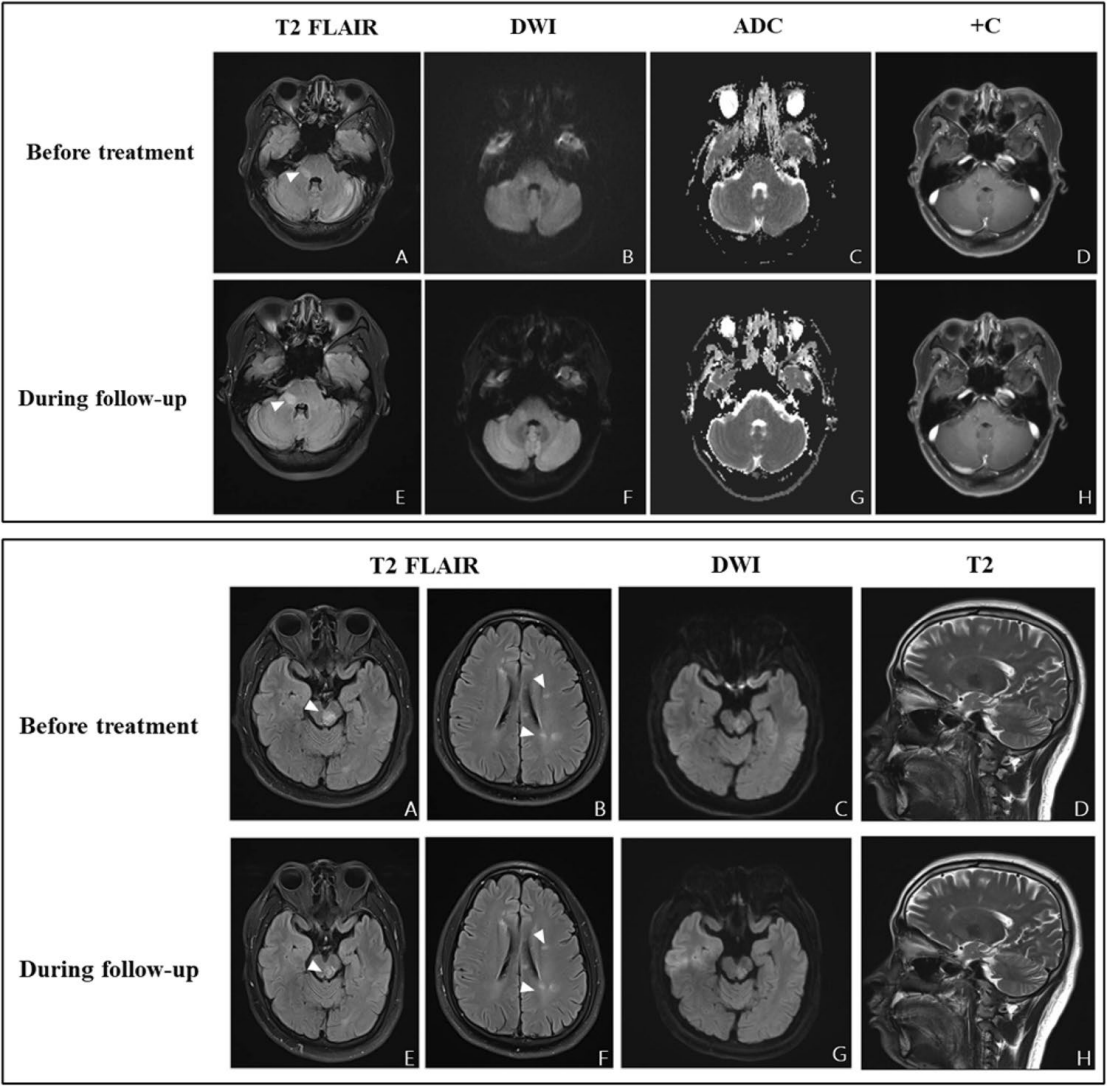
Up panel: Brain MRI of Patient 1. A–D show brain imaging examined before treatment, and E–H show imaging during follow-up period. A, E Hyperintensity in the right brachium pontis on T2-FLAIR; slightly increased intensity of the lesion on DWI (B, F) and ADC imaging (C, G). Punctate gadolinium enhancement of the lesion was observed on enhanced scan (D, H). Down panel: Brain MRI of Patient 4 A–D show brain imaging examined before treatment, and E–H show imaging during follow-up period. A Hyperintense lesion in the midbrain on T2-FLAIR and the signal was reduced during the follow-up (E); C, G The lesion presented hyperintense signal on DWI (A, E); B, F Hyperintense lesions distributed along the ventricle in axial imaging and sagittal imaging on T2W (D, H)
